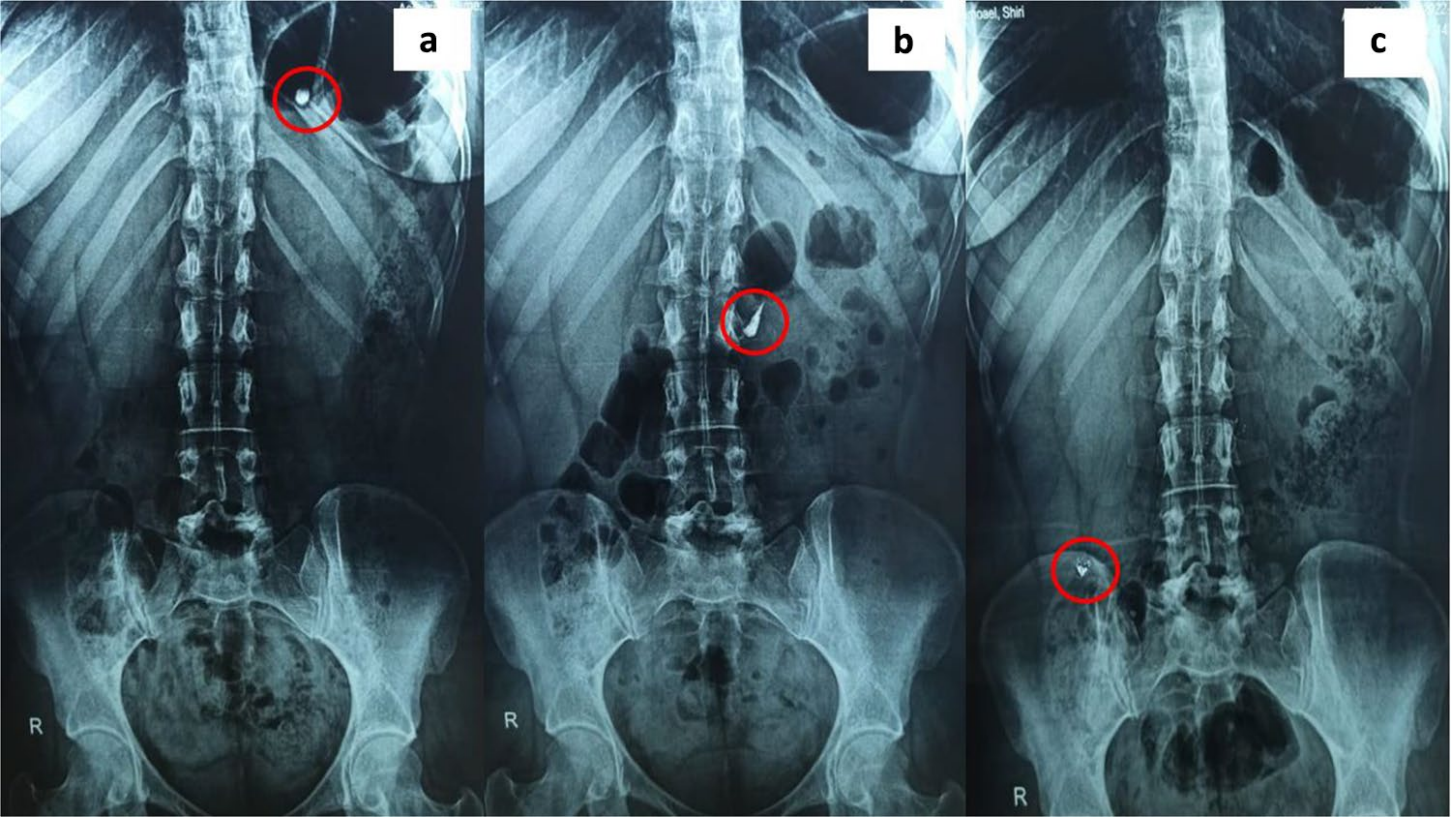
X-ray imaging of BaSO_2_-ZNPs-loaded colon-targeting capsules after oral administration in human volunteers. a 0.5 h post-dose, b 3 h post-dose, and c 5 h post-dose
